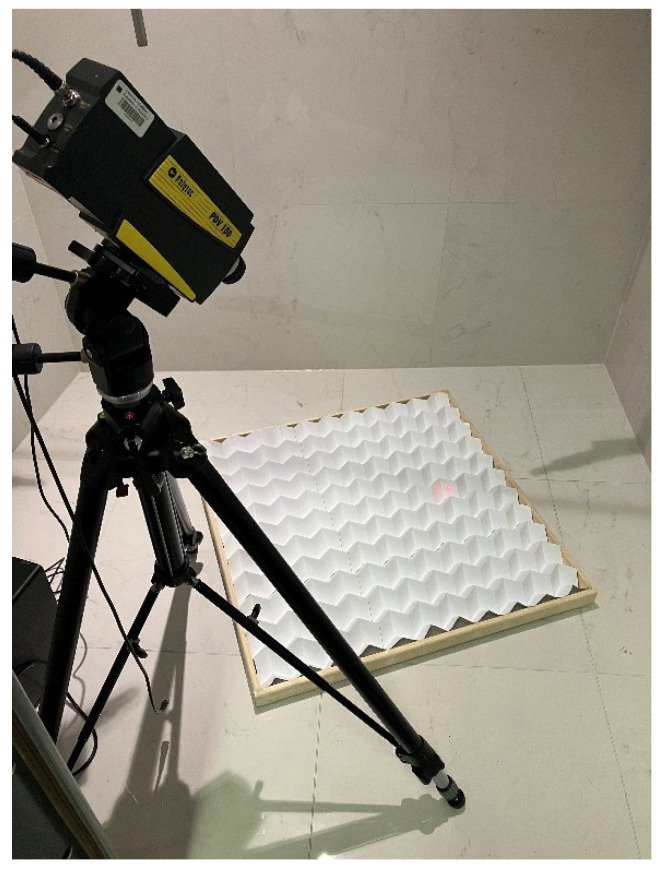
The non-contact vibration velocity measurements performed with a 1D laser Doppler vibrometer Polytec PDV100.
